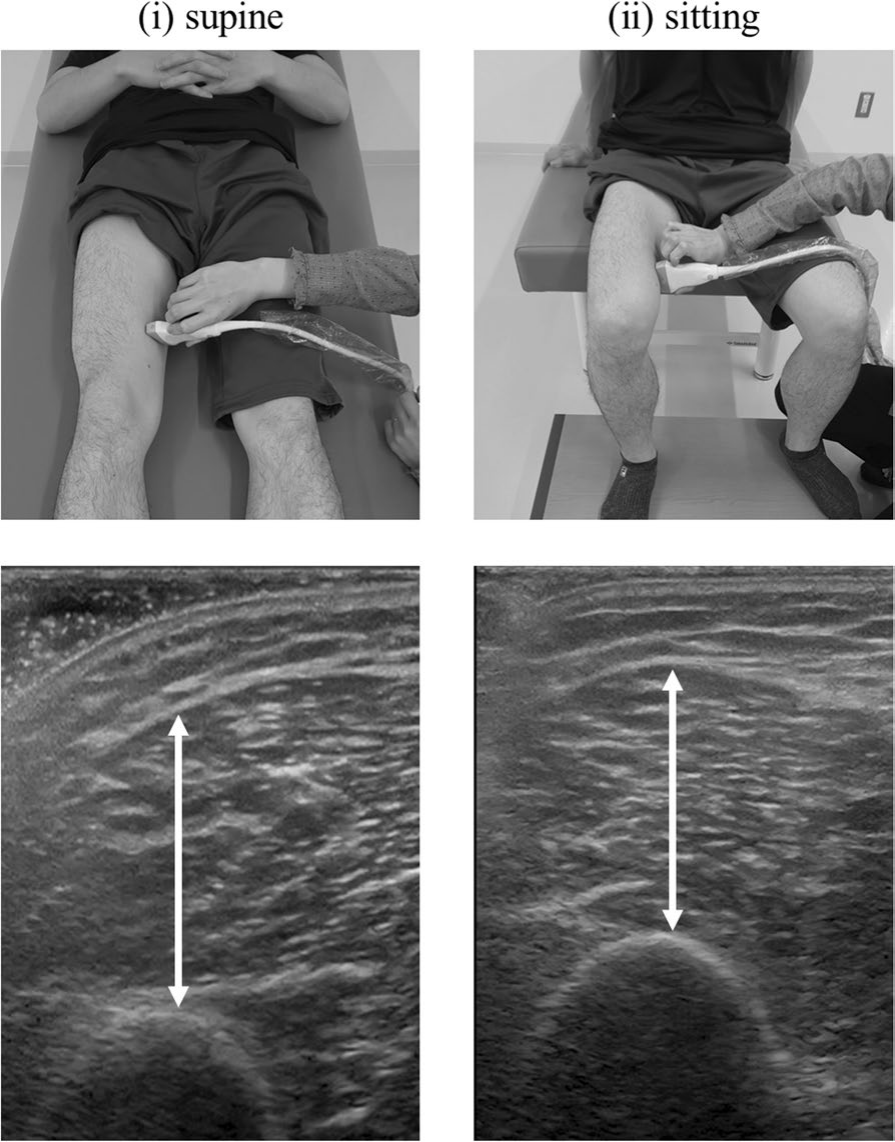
Measurement postures and representative ultrasound images of the vastus medialis captured in (i) supine and (ii) sitting postures. Ultrasound images of the vastus medialis in supine and sitting postures of young men who participated this study
